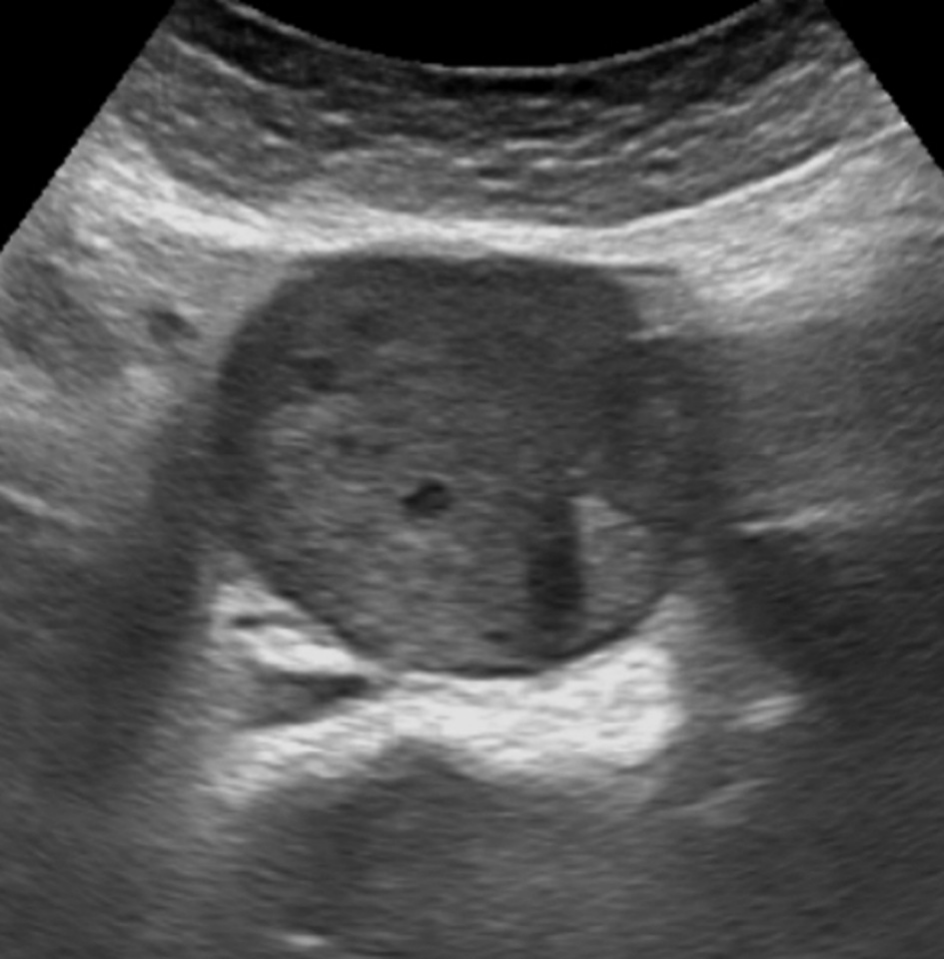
Abdominal ultrasound demonstrates a round soft-tissue mass with heterogeneous echogenicity and small areas of cystic degeneration.
